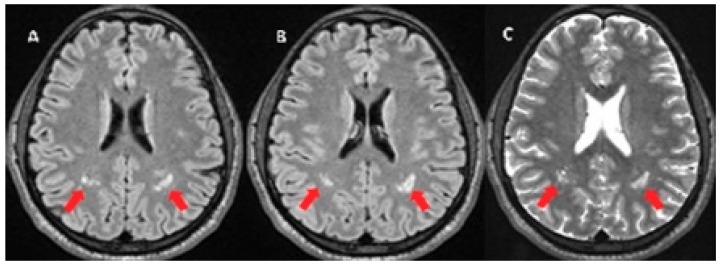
MRI with white matter hyperintensities (red arrow) in FLAIR (fluid attenuated inversion recovery) (A,B), and on T2 weighted images (C).
